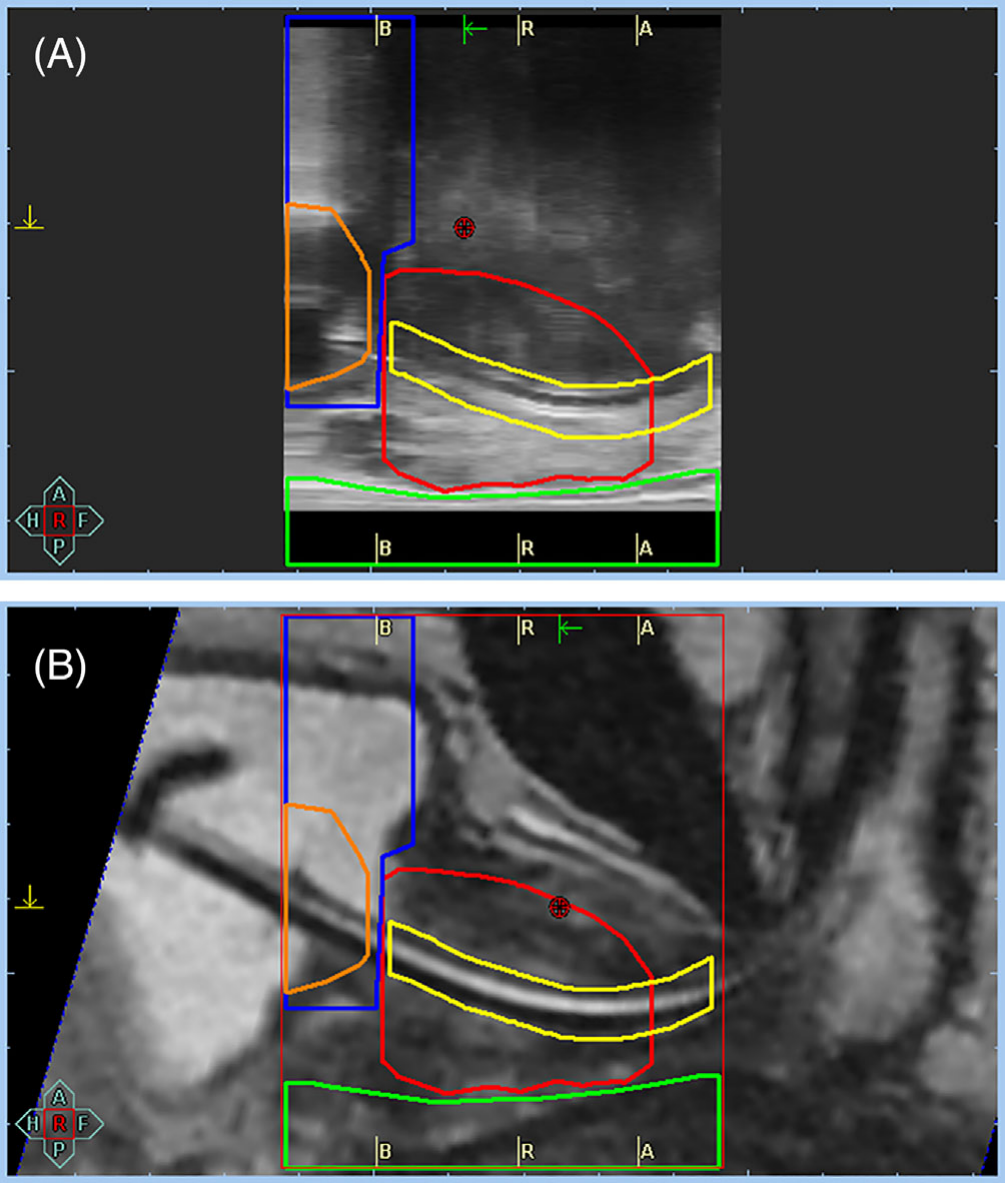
Image fusion with (A) ultrasound (US) image acquisition prior to interstitial catheter implantation and (B) magnetic resonance imaging (MRI) acquired on day of brachytherapy session with urethral catheter in place. Urethral catheter's balloon serves as a mark indicating the vesicourethral junction, a point easily identifiable both on US‐ and MRI‐images. MRI images assist in visibility of the prostatic base and apex. *blue contour = urinary bladder, red = prostate, yellow = urethra, green = rectum, orange = catheter balloon
